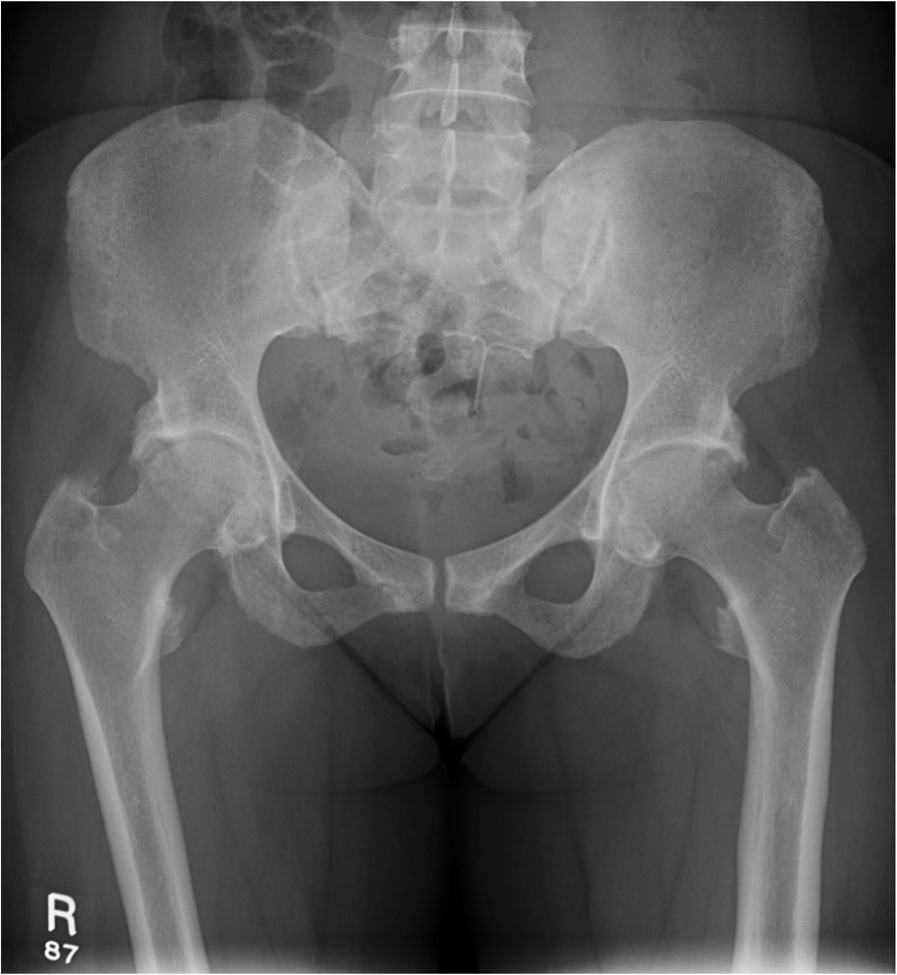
The pelvic radiograph showed mild degree of osteoarthritis in both hip joints with femoro-acetabular impingement (cam and pincer impingement)
